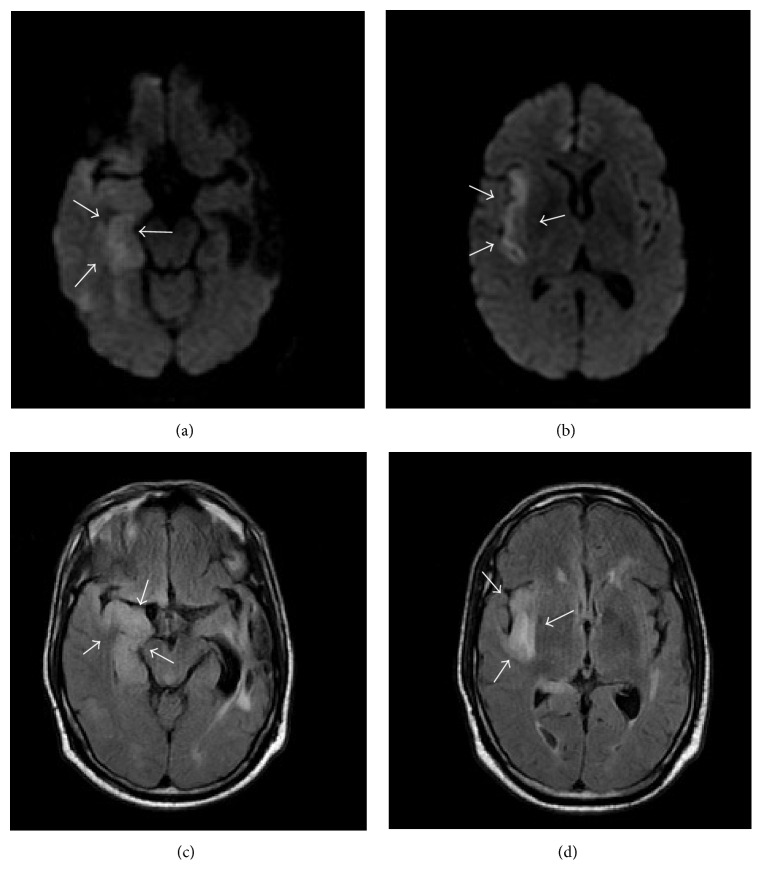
(Day 2) ((a) and (b)) noncontrast MRI of the brain: Diffusion Weighted Imaging (DWI) demonstrates increased signal in the right temporal lobe (a) and right insula (b), consistent with cytotoxic edema. Restricted diffusion is less intense as compared to infarction. These imaging findings in combination with clinical scenario are highly suggestive of herpes simplex encephalitis. ((c) and (d)) Noncontrast MRI of the brain: FLAIR sequence demonstrates asymmetrical involvement of the right medial temporal lobe (c) and the insular cortex (d). Unlike in middle cerebral territory infarct, basal ganglia is spared. Arrows in (a) refer to increased signal in right temporal lobe, in (b) increased signal in right insula, in (c) FLAIR sequence demonstrating involvement of right medial temporal lobe, and in (d) FLAIR sequence demonstrating involvement of right insular cortex.
